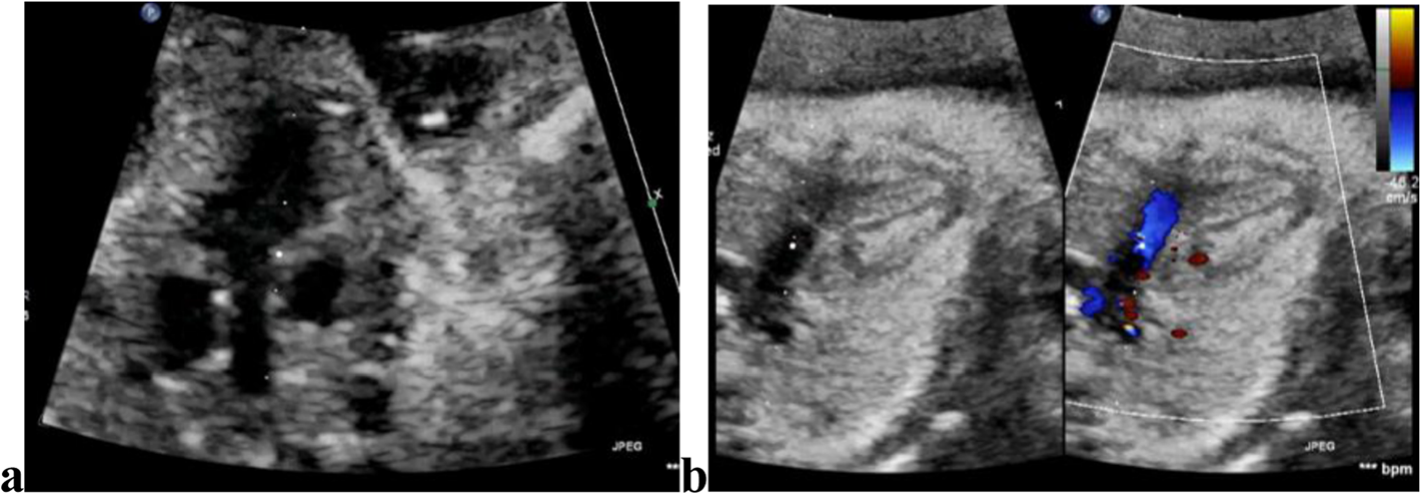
a Shows left ventricle outflow tract view by 2D imaging in a case 13-week gestation. b Shows right ventricle outflow tract by both 2D and color Doppler imaging in a case 11-week gestation
